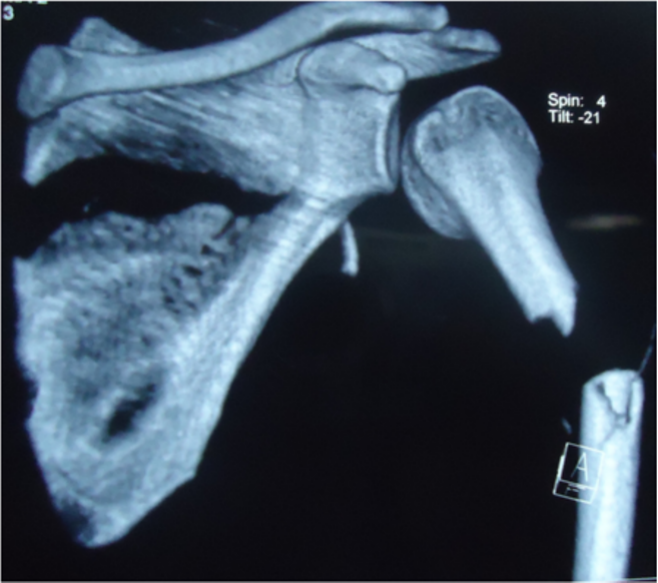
Computed tomography (virtual reconstruction) outlining the scapular fracture pattern.
